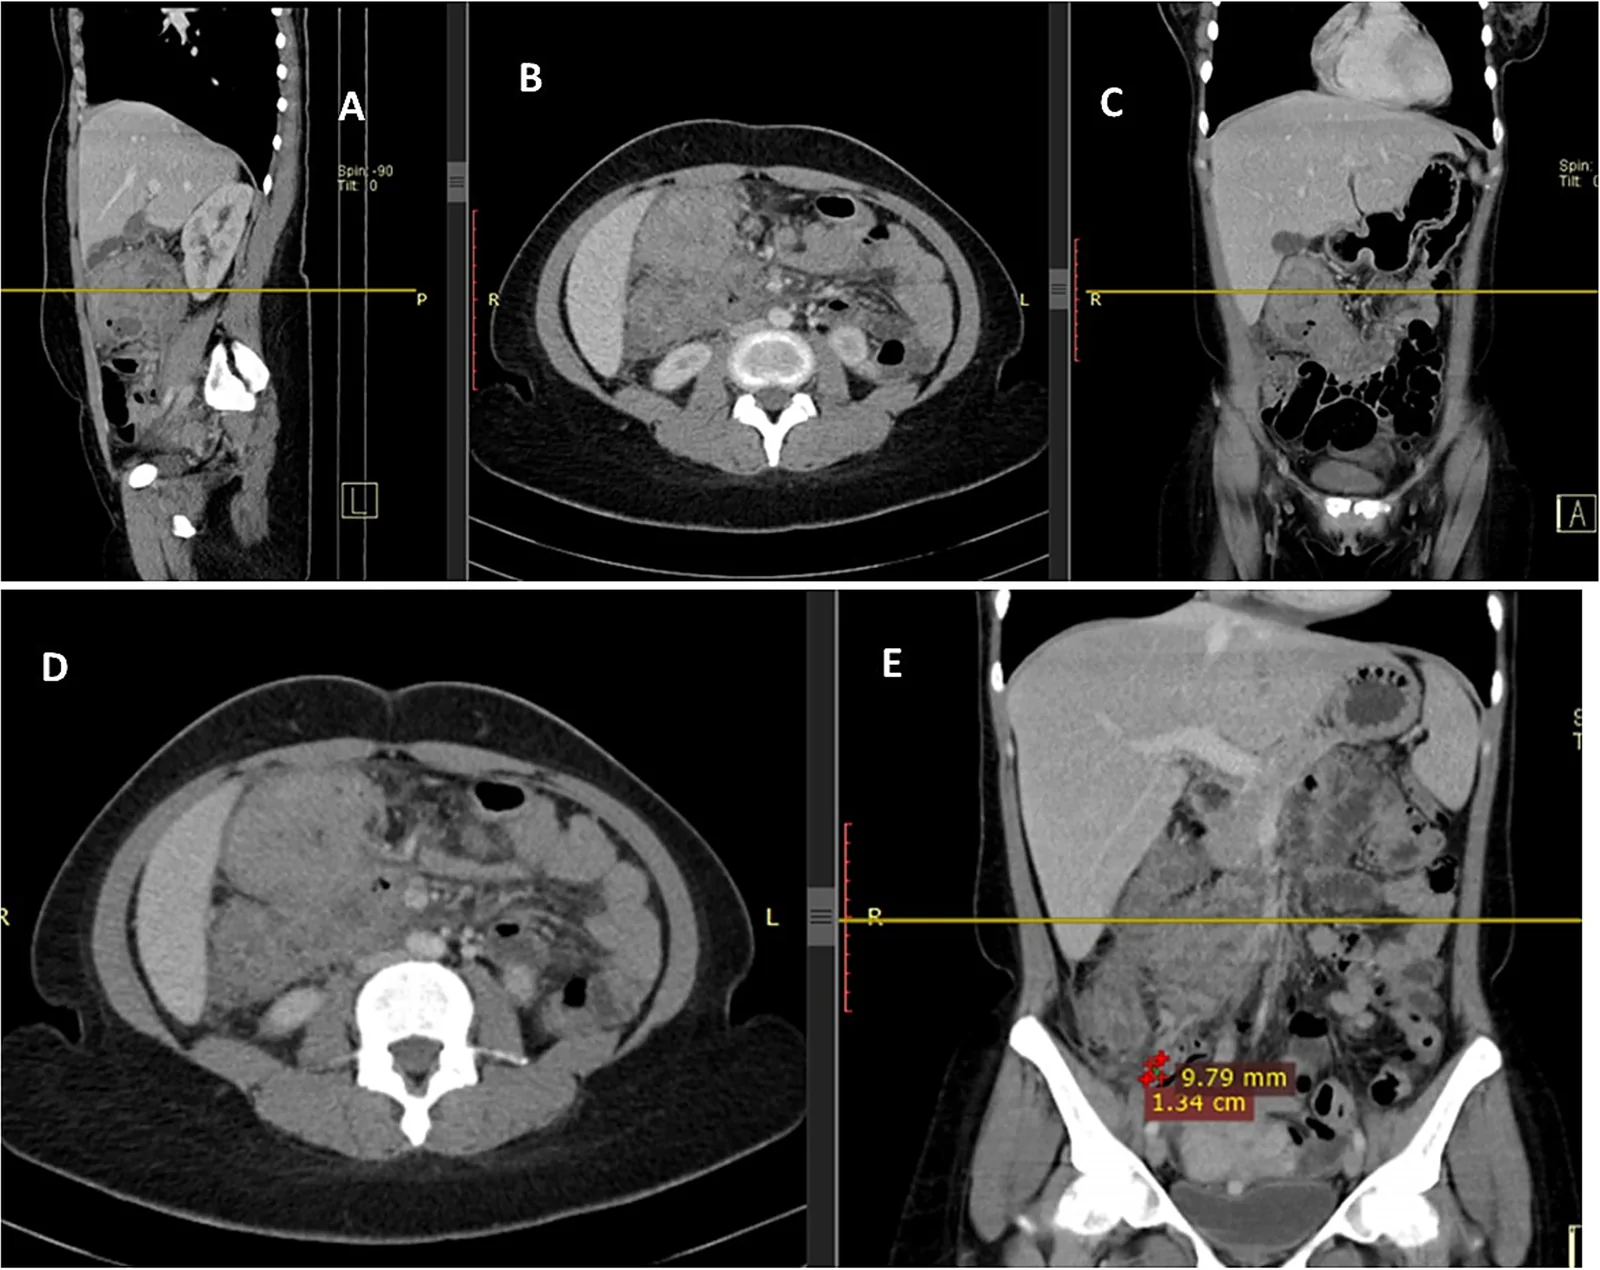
Contrasted abdominal-pelvic CT scan images; A (sagittal), B (axial) and C (coronal) planes showing a large, 7.04 × 5.2 × 8.58 cm sized heterogenous enhancing soft tissue lesion centered at the hepatic flexure abutting the distal D2, extending to both distal ascending colon and proximal transverse colon with perilesional mesenteric fat stranding. Images D (axial) and E (coronal) depicts tumour infiltration to middle colic and right colic branches, as well as abutment of the infrarenal inferior vena cava and inferior mesenteric vein. Multiple perilesional, mesenteric and retroperitoneal lymphadenopathy also noted. There were no pulmonary, hepatic or osseous metastasis noted.
